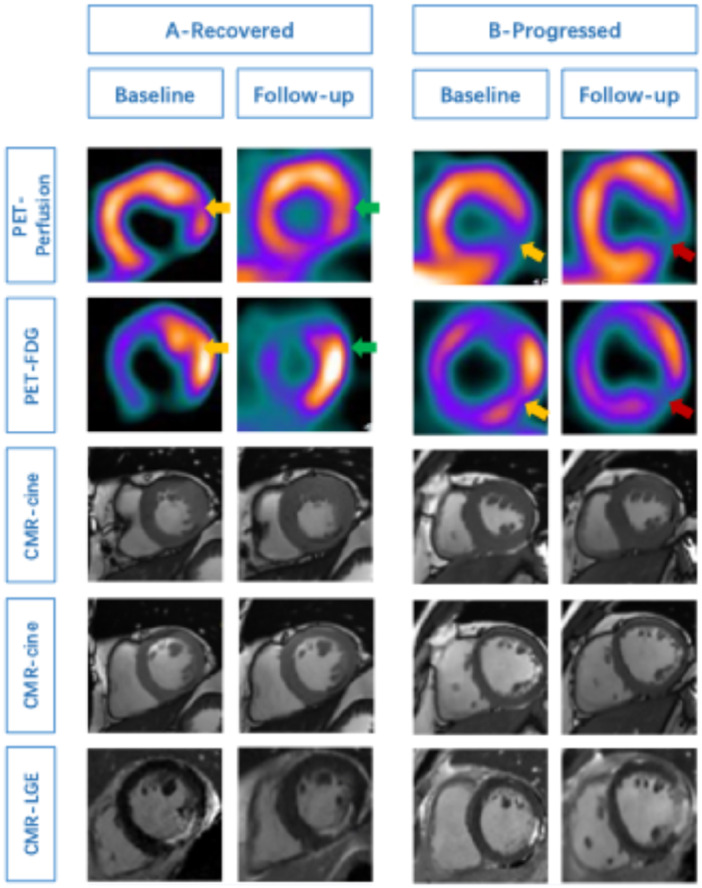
Representative PET/MR image of 2 separate patients. (A) Short‐axis images obtained from 2‐month and 1‐year scans of a 49‐year‐old male who initially presented with inferior STEMI and underwent PCI to his RCA. Hypoperfused myocardium was observed in the mid inferolateral wall at baseline scan (yellow arrow), and recovered at follow‐up (green arrow), with evidence of ameliorated perfusion and improved segmental wall movement. (B) Short‐axis images obtained from 2‐month and 1‐year scans of a 43‐year‐old male who also initially presented with inferior STEMI and underwent PCI to his LCX. Hypoperfused myocardium was observed in the basal inferolateral wall at baseline scan (yellow arrow), and progressed to scar at follow‐up (red arrow), showing diminished metabolism and no significant improvement in segmental wall movement. FDG = fluorodeoxyglucose; GLS = global longitudinal strain; hs‐CRP = high‐sensitivity C‐reactive protein. LCX = left circumflex; LGE = late gadolinium enhancement; PCI = percutaneous coronary intervention; PET/MR = positron emission tomography/magnetic resonance; RCA = right coronary artery; STEMI = ST‐segment elevation myocardial infarction.
